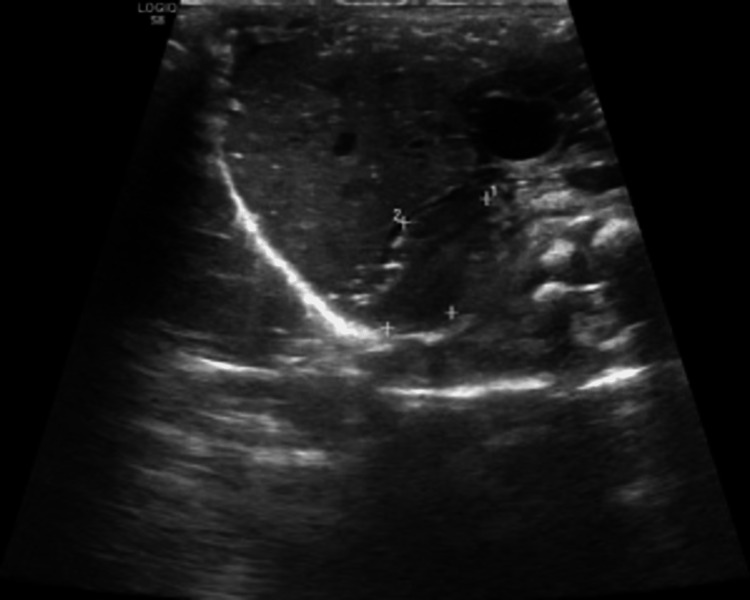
Abdominal ultrasound showing right suprarenal gland is enlarged (2.15 x 1.34 cm) with loss of corticomedullary differentiation.
